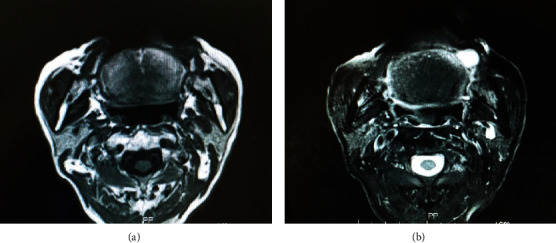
Magnetic resonance imaging of the tumor. (a) T1-weighted MRI showed a mass with low intensity in comparison with skeletal muscle tissue (yellow dot) while (b) T2-weighted MRI revealed a lesion with high intensity in comparison with adipose tissue (yellow dot).
